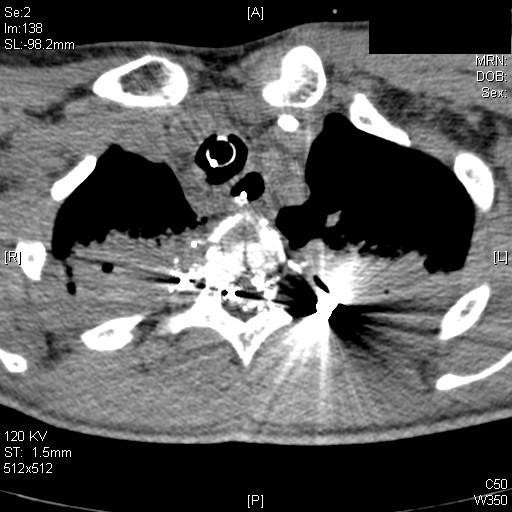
CT-scan: Spinal gunshot with destruction of the spinal cord. These injury caused a complete paraplegia and an unstable fracture of the vertebra.
